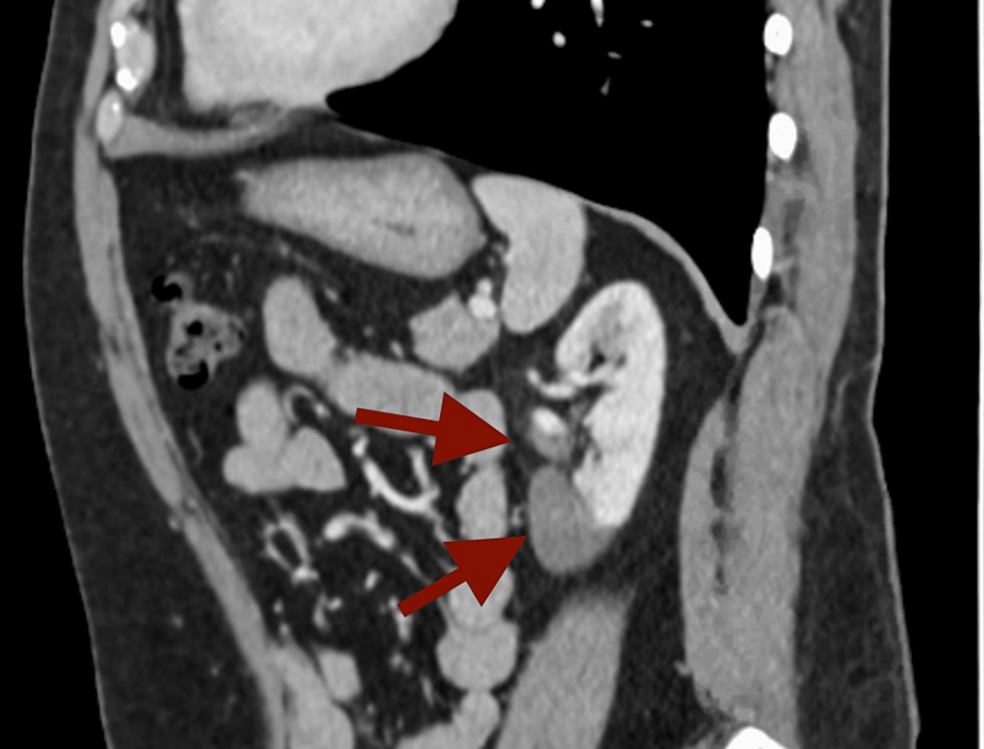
CT scan with contrast of the abdomen and pelvis depicting renal artery thrombosis with renal infarct in the mid to lower pole of the kidney
